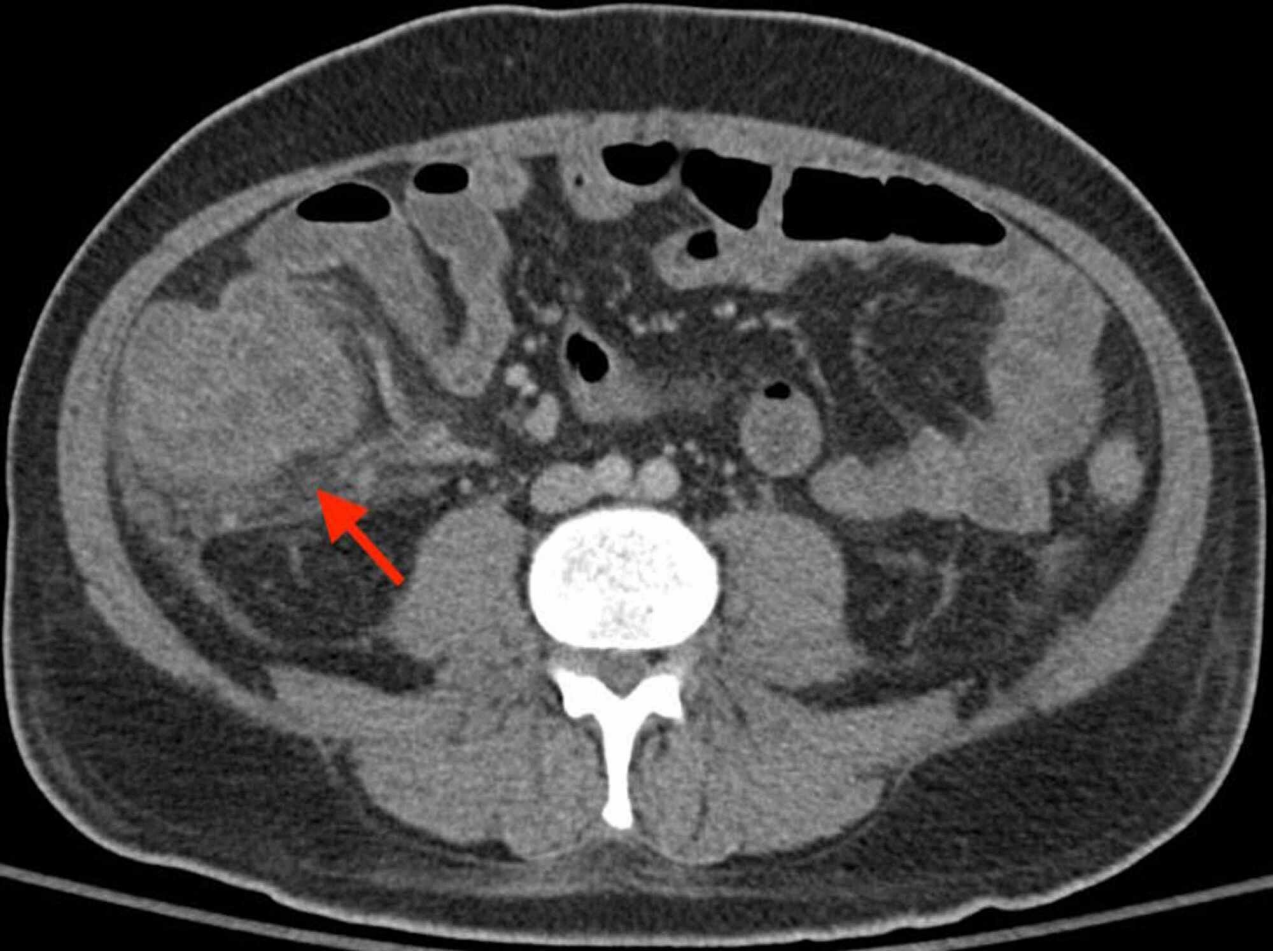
CT showing marked wall thickening involving the cecum and right hemicolon, consistent with neutropenic enterocolitis.
